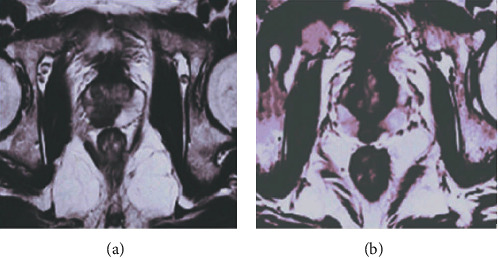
Comparison on MRI images of prostate cancer and benign prostatic hyperplasia. (a) Prostate cancer. (b) Hyperplasia of prostate.
